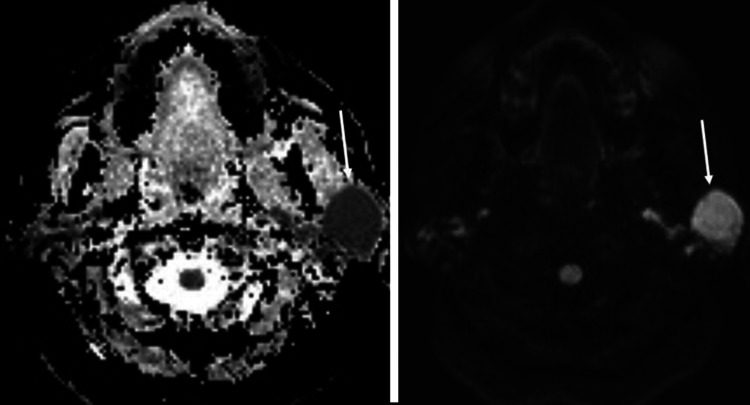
Axial ADC, left, and axial DWI, right, both at the level of the parotids. The ADC map shows a very low signal with a corresponding high signal on DWI of the left parotid mass (white arrows). This is consistent with significantly restricted diffusion. ADC: apparent diffusion coefficient; DWI: diffusion-weighted imaging
